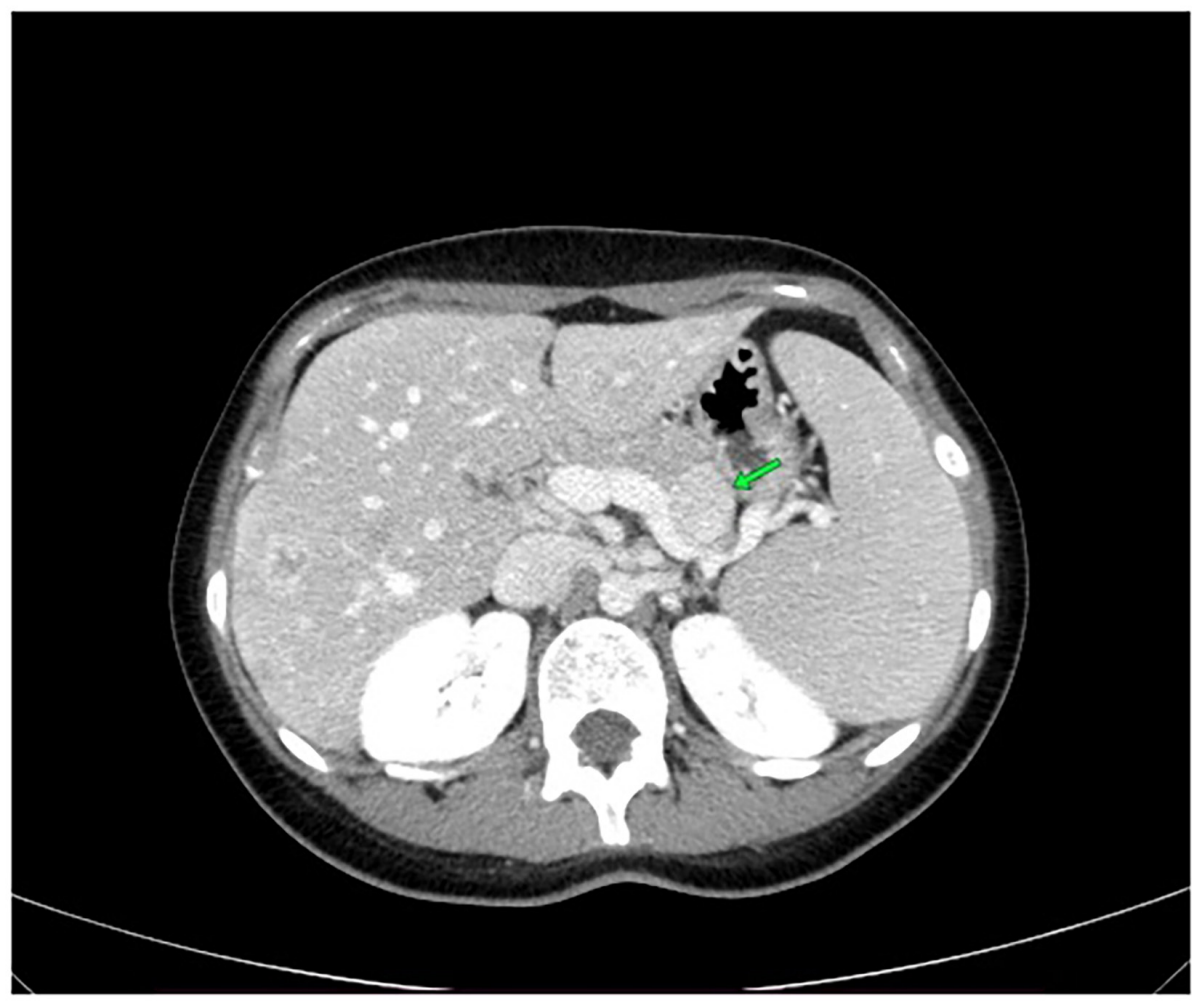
A 17‐year‐old female with metastasized pancreatic neuroendocrine tumor. CT image of the pancreatic mass showing a well‐defined heterogeneous mass in the tail.
